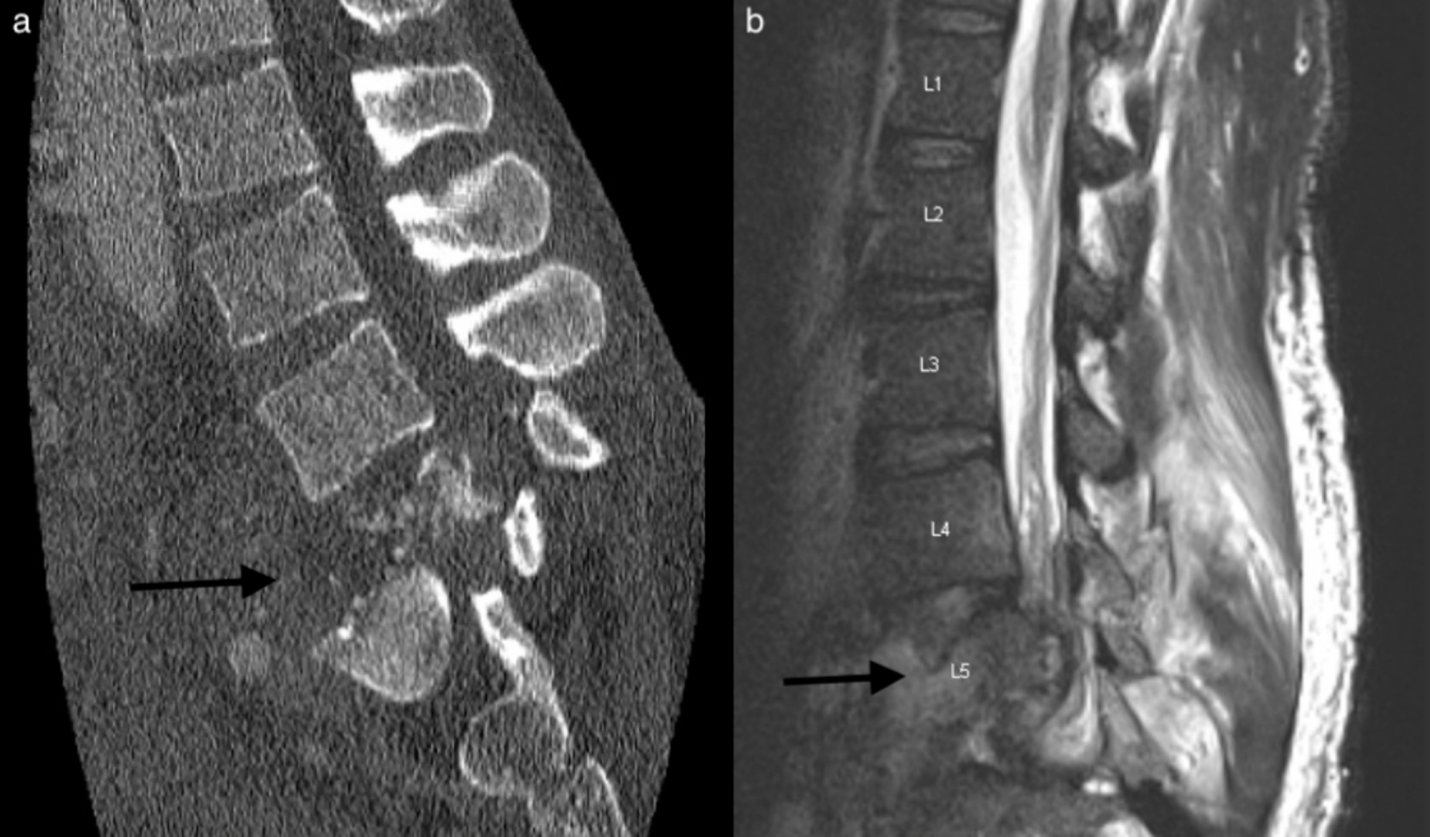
Preoperative CT and MRI of lumbar spine (a) CT lumbar spine in the sagittal plan demonstrating L5 burst fracture. (b) T2 lumbar MRI in the sagittal plane demonstrating severe destructive process of L5 with extensive epidural and soft tissue enhancement concerning for infection.
